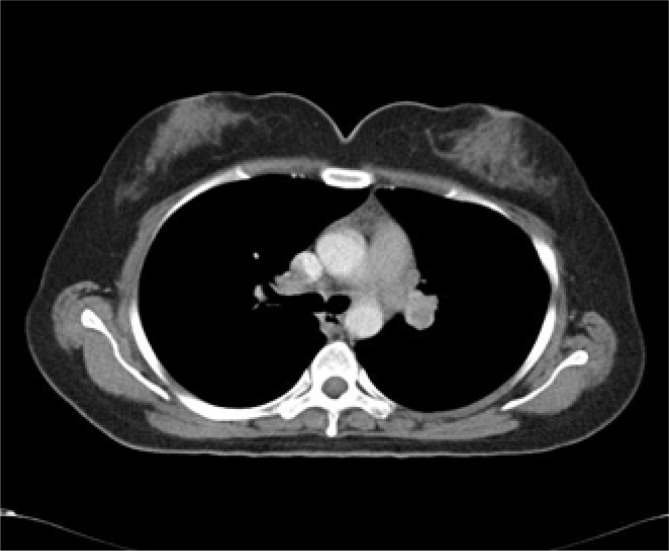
Thorax CT showed bilateral hilar lymphadenopathy (grade 1 sarcoidosis) – 3months after delivery.
